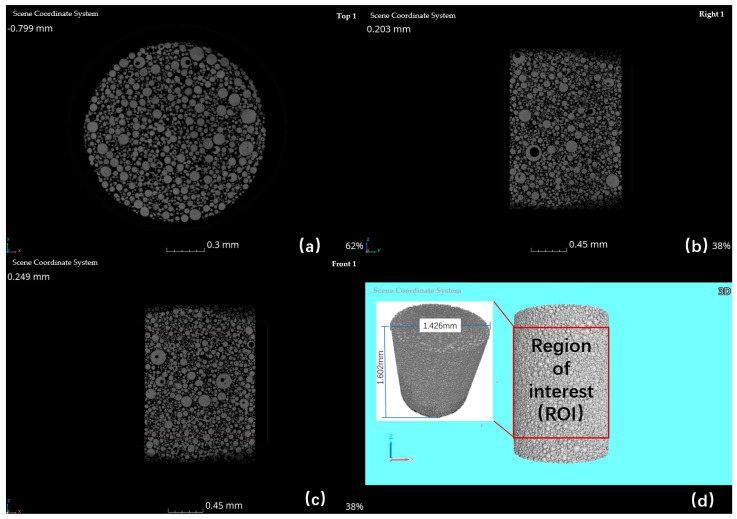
2D CT image of Ti6Al4V powders: (a) XY−plane; (b) YZ−plane; (c) ZX−plane; (d) 3D model of the powder sample reconstructed by VGstudioMAX 2023.2, and the region of interest (ROI).
